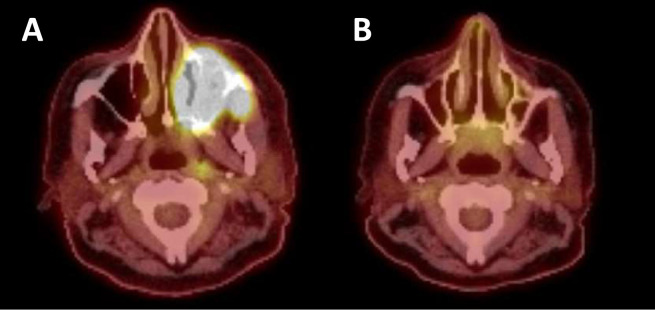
A) Positron emission tomography – computed tomography (PET-CT) scan demonstrating extramedullary disease prior to bridging and CAR T cell therapy B) PET-CT scan demonstrating response to bridging and CAR T cell therapy.
